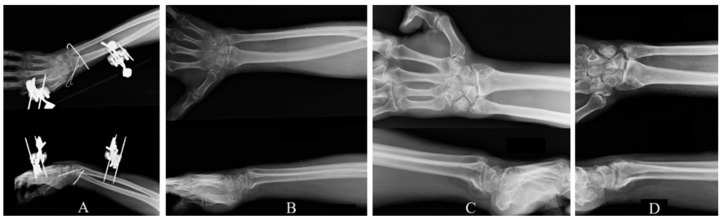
AP and Lateral X-ray views of right wrist at (A): 2-month, (B): 12-month, (C): 2-year, (D): 4-year follow-ups.
